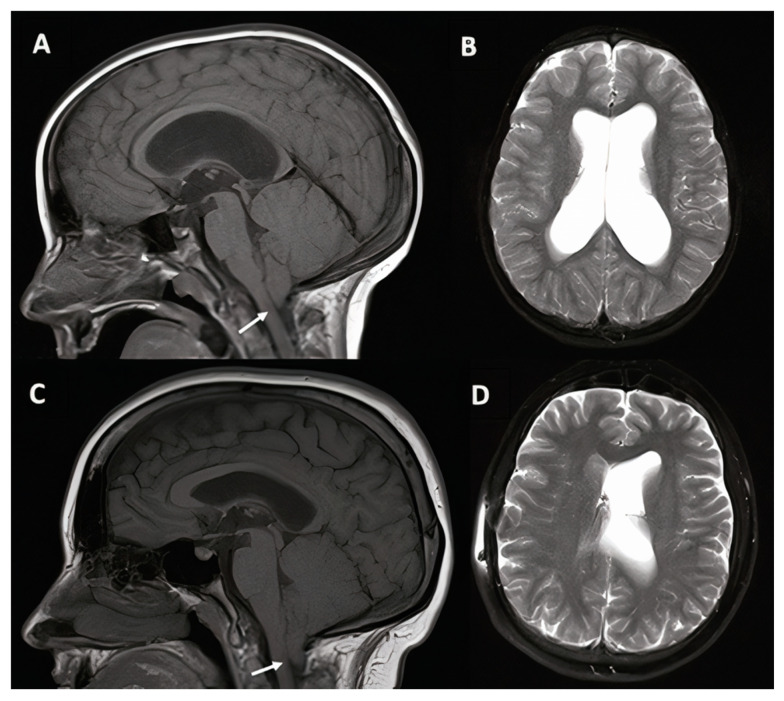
6-year-old female patient with Chiari I malformation (case #1). Pre-operative (A,B) and 10 years post-operative (C,D) T1-weighted midsagittal and T2-weighted axial magnetic resonance imaging (MRI). Inferior displacement of the cerebellar tonsils below the foramen magnum consistent with Chiari malformation is indicated by the white arrows.
